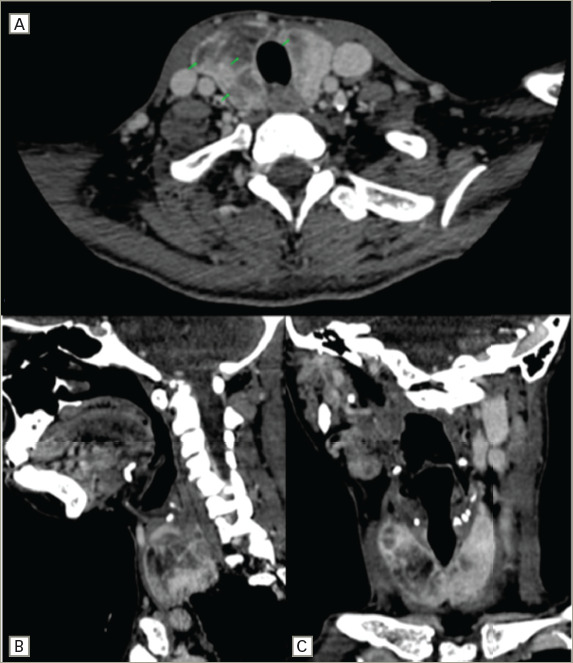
Pre-operative cervical computed tomography scan with contrast
